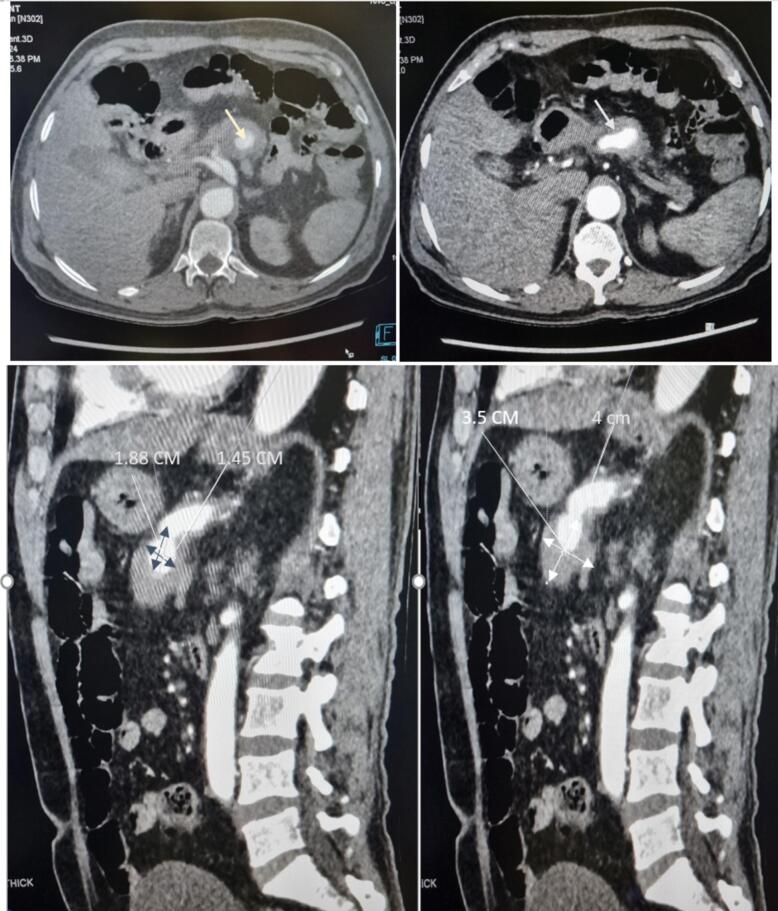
Contrast computed tomography showing outpouching of approximate 1.88 cm ∗ 1.45 cm and hypo-dense area of 3.5 cm ∗ 4 cm around outpouching.
